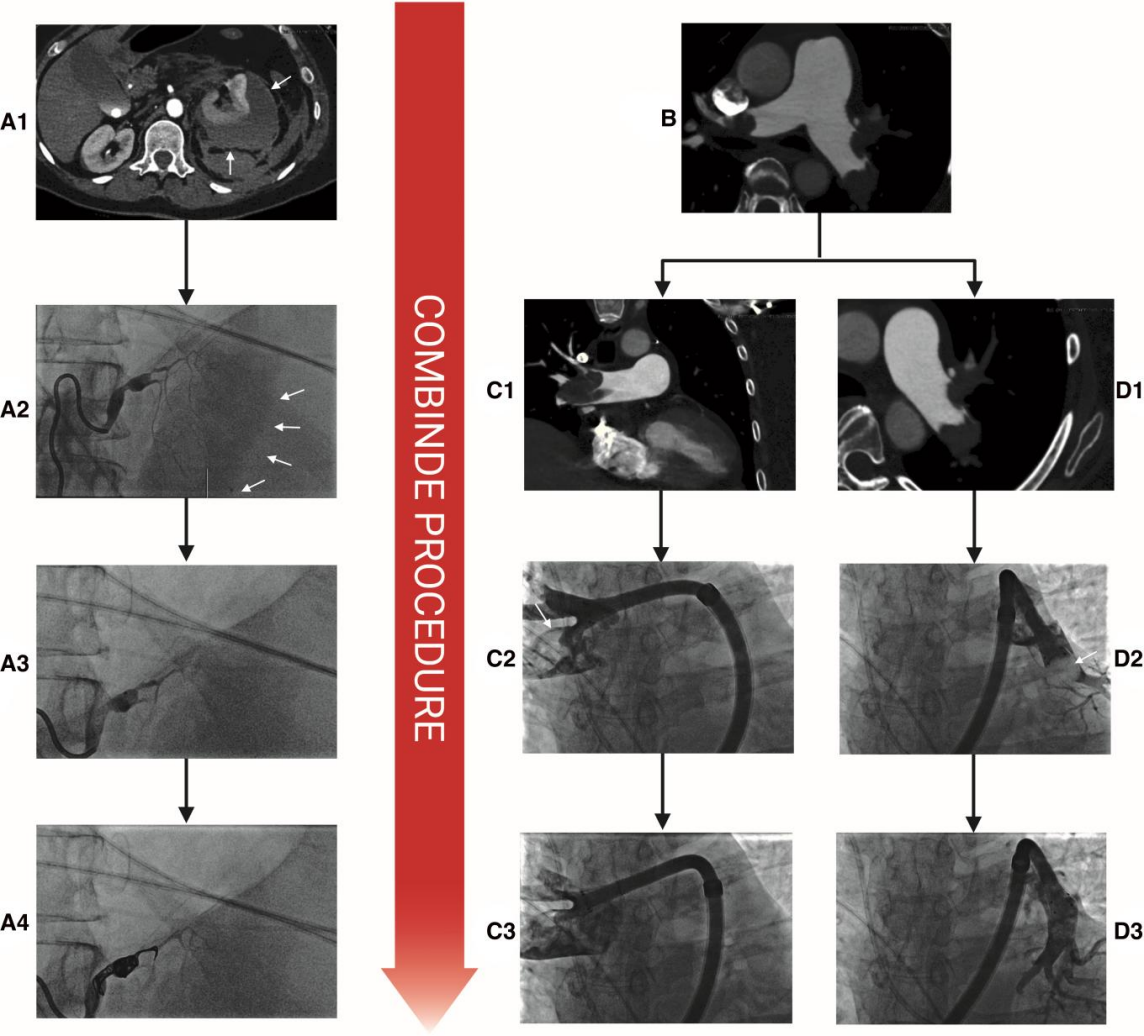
A1: CT scan of kidney haemorrhage (arrows pointing at haematoma), A2: renal angiography with diffuse microbleeding (white arrows), A3: renal angiography after injection of EmboSphere, A4: final renal angiography after embolization and coiling. B: CT scan showing bilateral central pulmonary embolism. C1: CT scan of the right pulmonary artery showing a large central thrombus, C2–3: pulmonary angiography showing thrombus (white arrow) before aspiration (C2) and after (C3) in the right pulmonary artery. D1: CT scan of the left pulmonary artery showing a large central thrombus, D2–3: pulmonary angiography showing thrombus (white arrow) before aspiration (D2) and after (D3) in the left pulmonary artery. Created in BioRender. Trøan, J. (2026) https://BioRender.com/ewu94n3.
